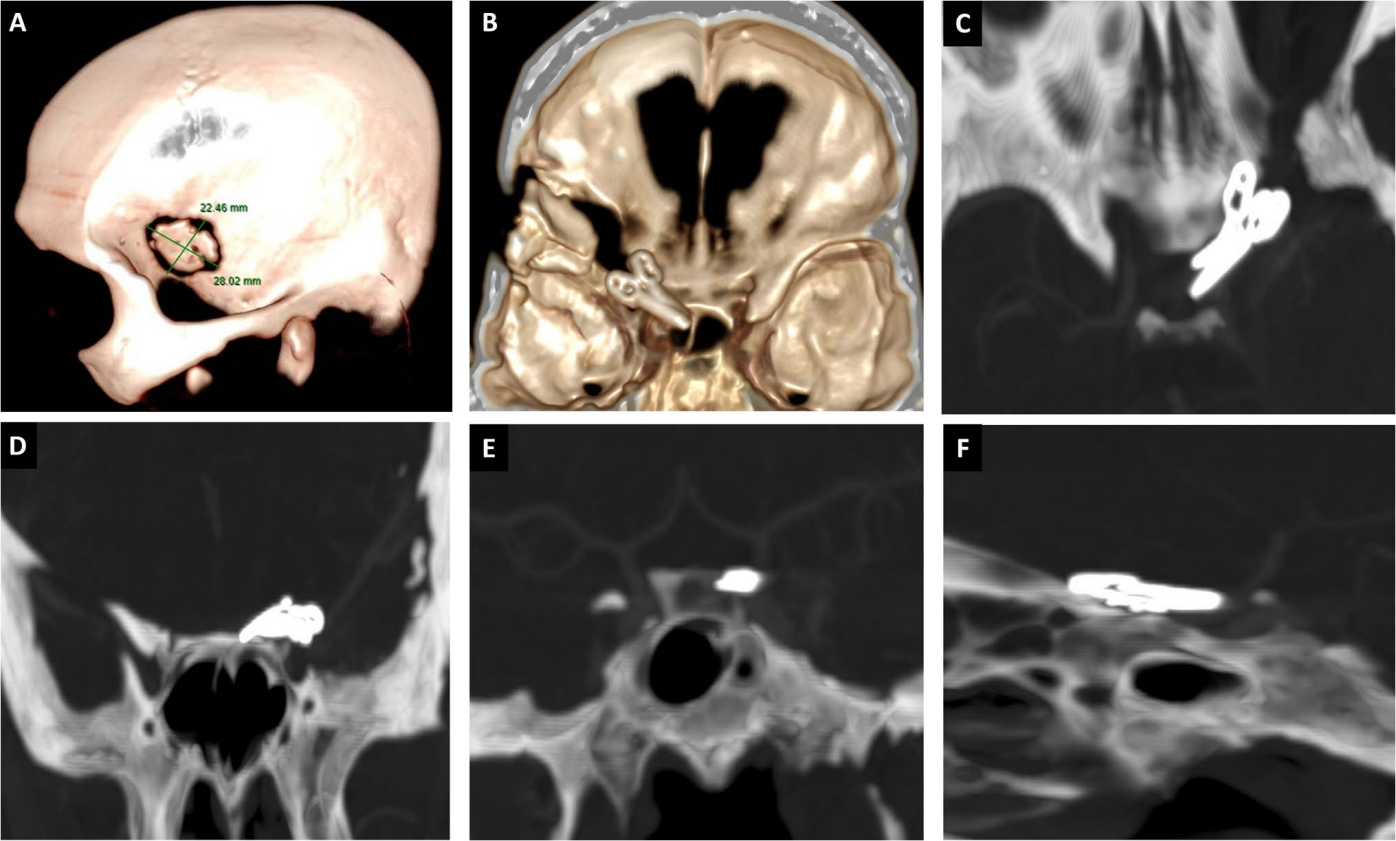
A 22 × 28-mm left keyhole sphenoid ridge craniotomy. B An extensive left sphenoid wing and APC removal are shown in the postoperative 3D CT bone reconstruction. The dorsal paraclinoid and carotid cave aneurysms were clipped with two 11-mm straight clips and one 9-mm 45º angled clip. The axial (C), coronal (D, E), and sagittal (F) postoperative CT angiography windows show the clip’s position, absence of aneurysms, and permeable distal flow in the ICA
